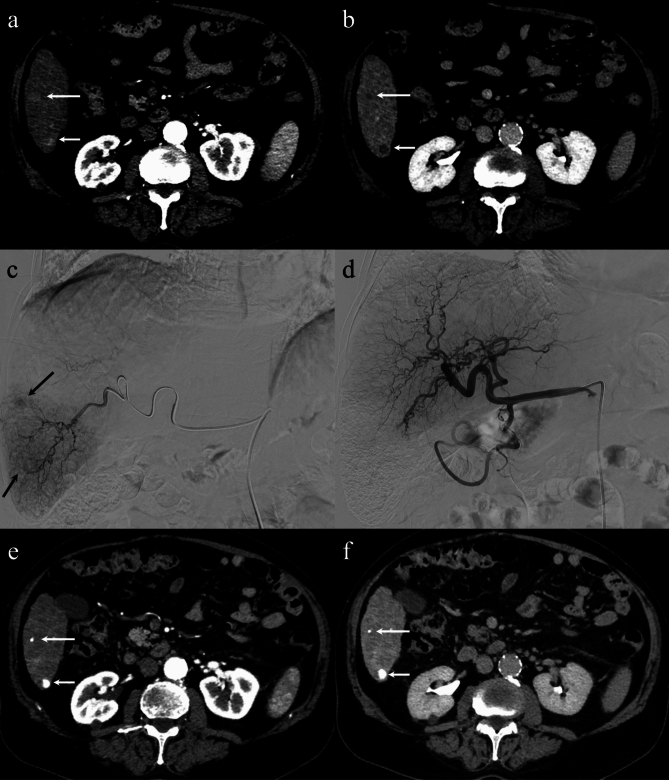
Contrast-enhanced computed tomography (CT) imaging shows the two nodular lesions of 13 mm and 10 mm within segment 6 of the liver, exhibiting arterial hypervascularization (arrows in a) and portal-venous washout (arrows in b), consistent with hepatocellular carcinoma (HCC). The angiographic study, in the parenchymal phase, confirms the two hypervascular nodules (arrows in c). After transarterial chemoembolization (TACE), the occlusion of the tumor-feeding vessels is demonstrated together with the patency of the remaining hepatic arteries (d). Contrast-enhanced CT performed 3 months post-TACE demonstrates the homogeneous accumulation of the Lipiodol in both lesions, with no signs of relapse in the arterial and portal-venous phases (arrows in e and f)
